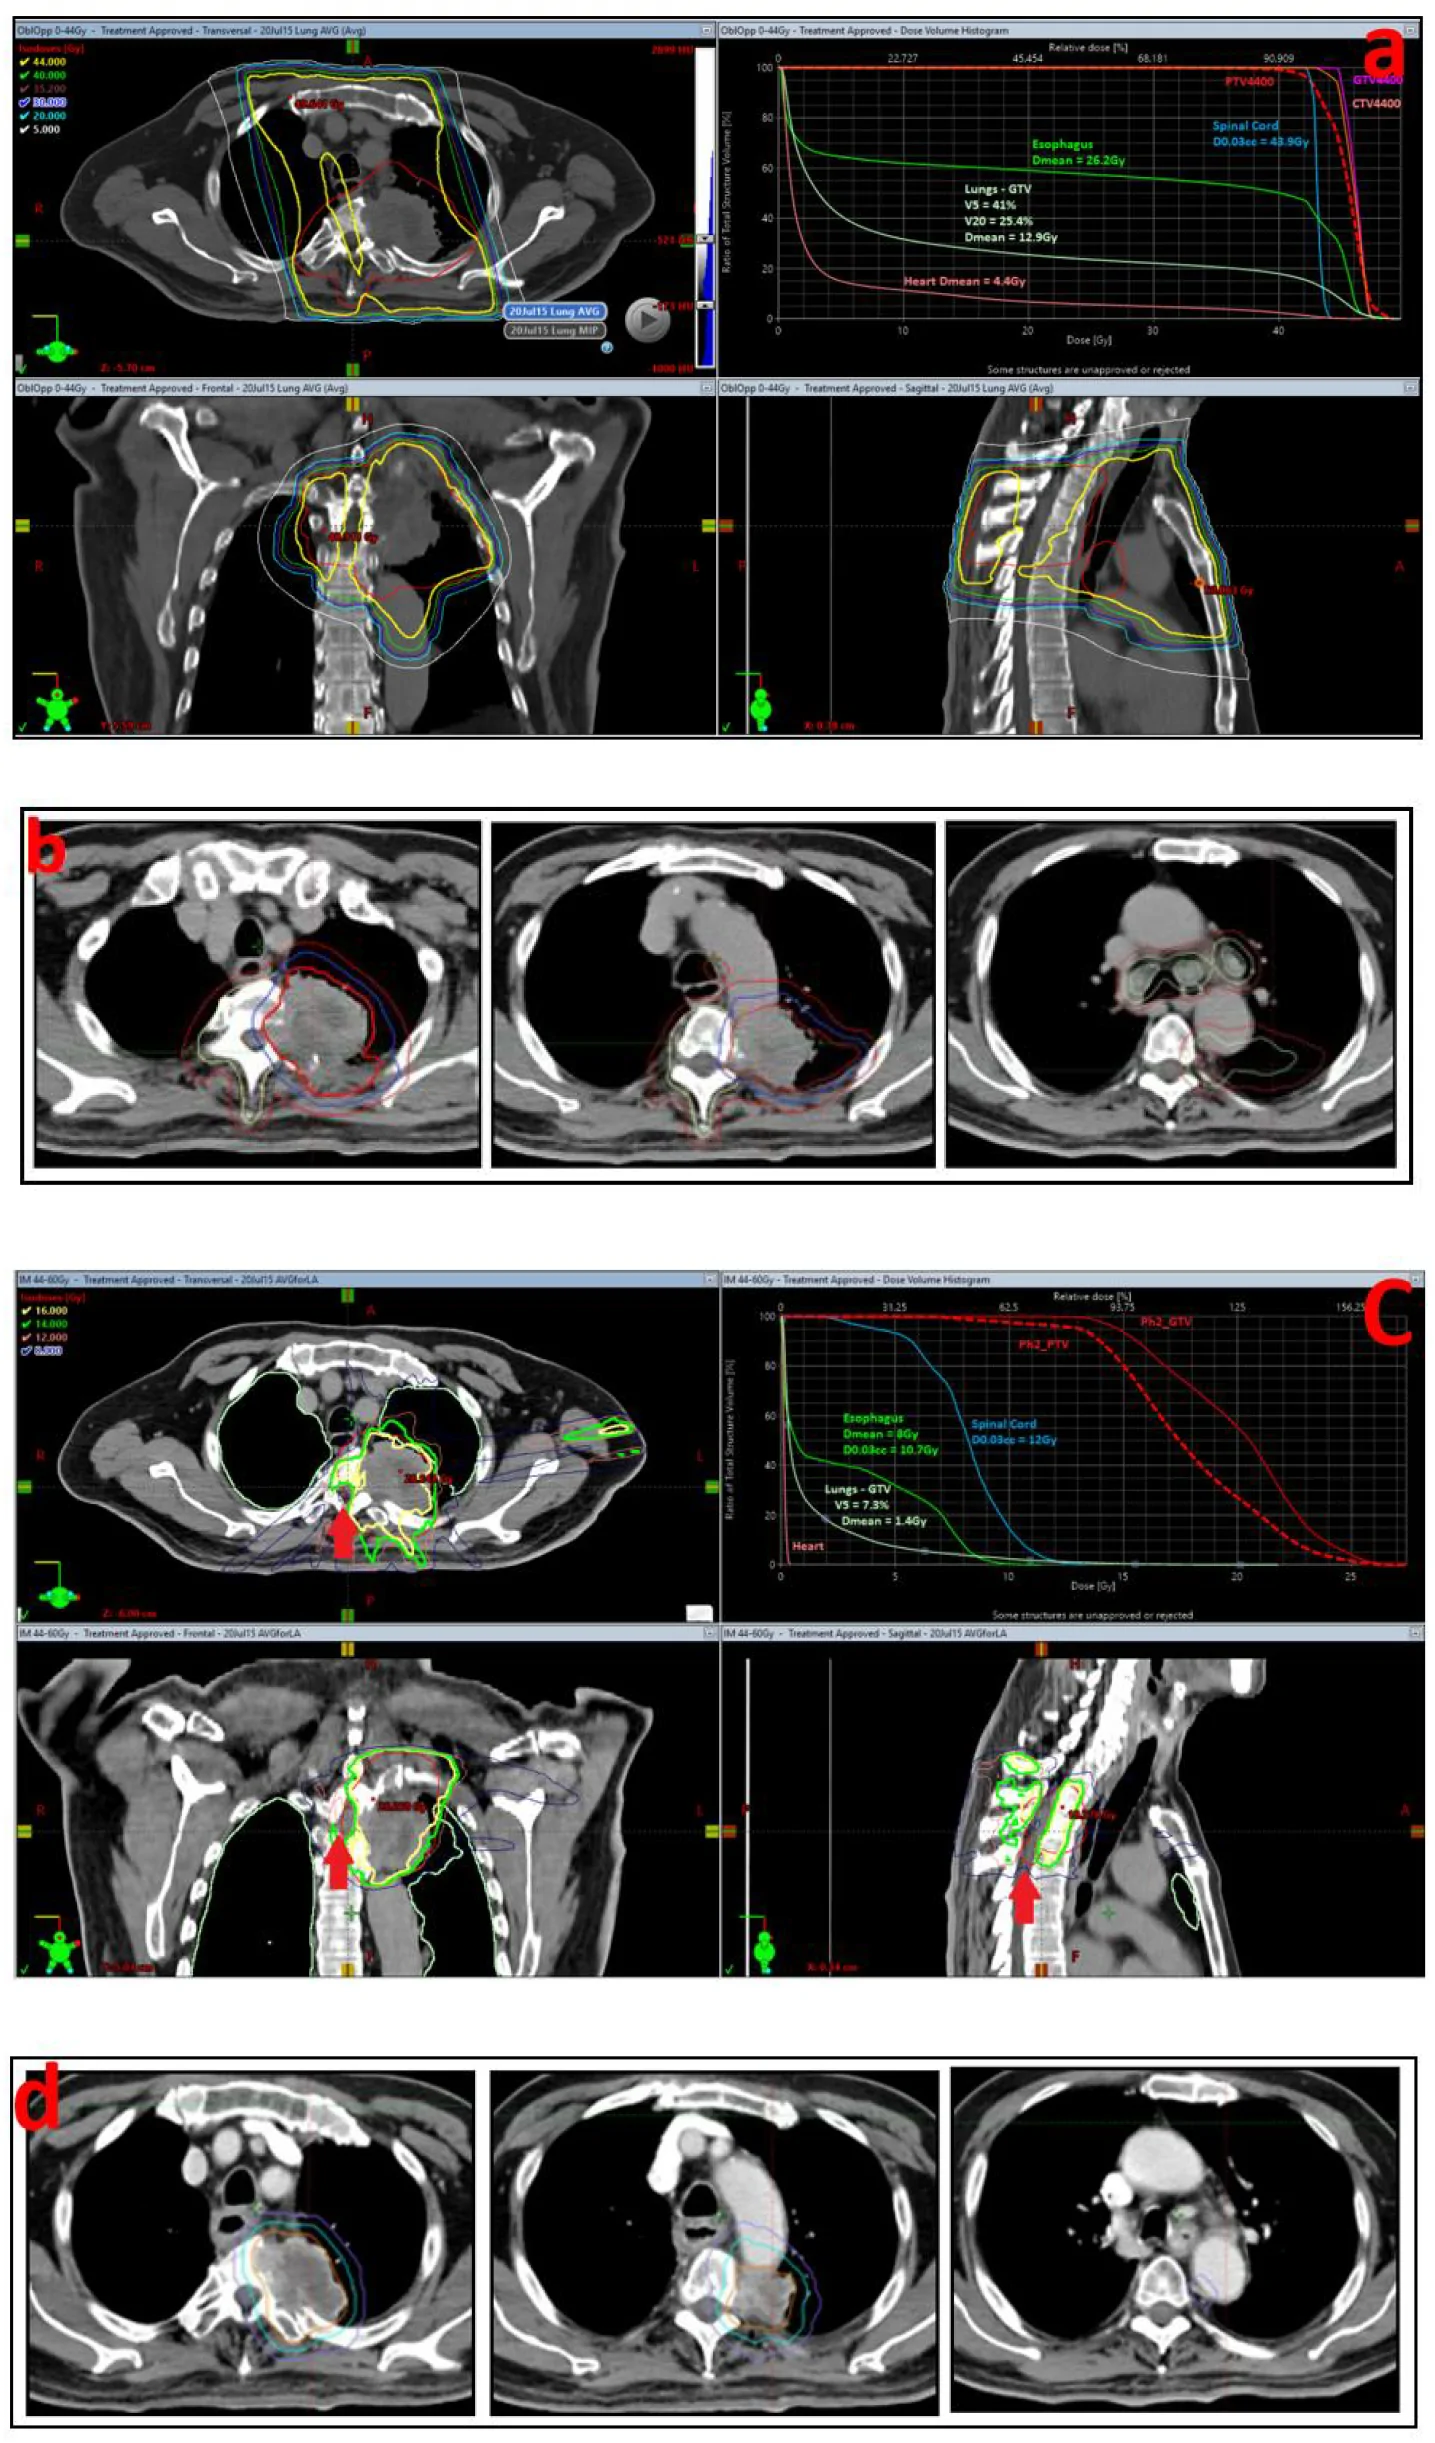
Isodose distributions of 1st 44 Gy and the adaptive radiotherapy plan. The panel (a) shows the radiation dose distribution of the first 44 Gy, on transverse, coronal, and sagittal views. The Phase I Clinical Target Volume (CTV) included the left upper lung tumors with a 5 mm margin from GTV, tumor involved vertebral bodies, level 4R/L lower paratracheal the mildly FDG avid nodes, level 5 a/p window node, and left level 10 hilar nodes (b). The isodose distribution of the adaptive phase shows adaptive boost dose on the primary tumor (c) and the Phase II CTV of the adaptive radiotherapy target included only the left upper gross lung tumors with a 5 mm uniform margin (d), without inclusion of the nodes.
